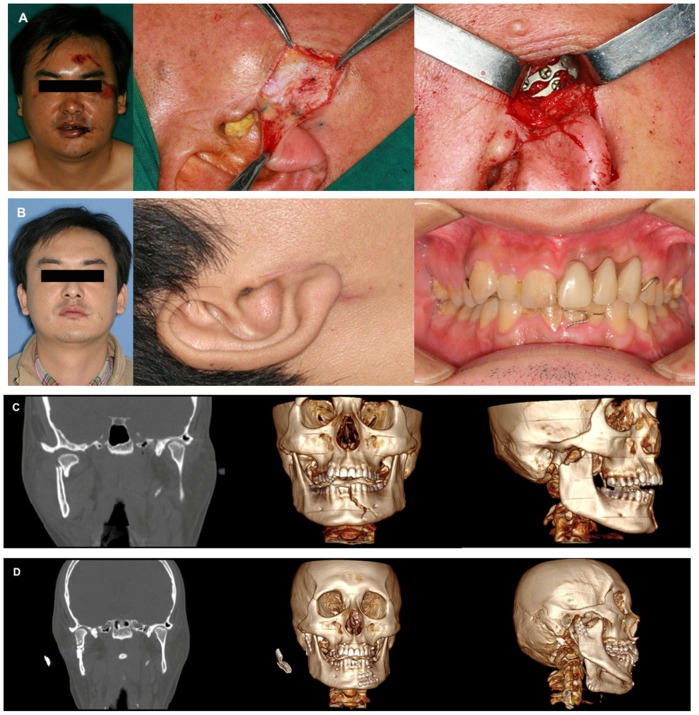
Photographs show a 33-year old male patient undergoing open reduction and rigid internal fixation of right condylar fracture 5 days after trauma. A: Pre-operative facial appearance and rigid internal fixation; B: Post-operative facial appearance and occlusion; C: Pre-operative CT scan and 3D reconstruction images; D: PT scan and 3D reconstruction images taken at follow-up.
